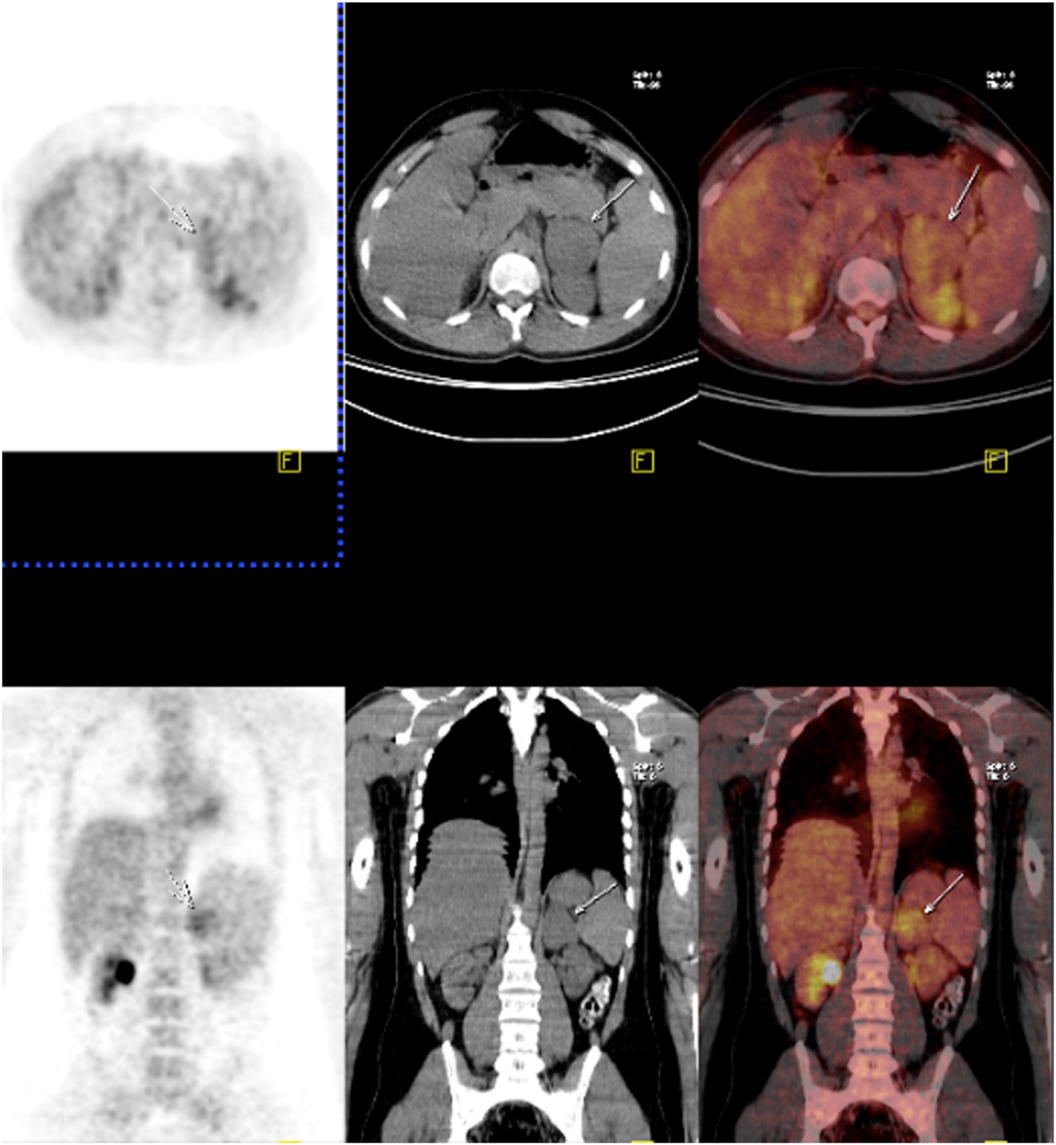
Positron emission tomography and computerized tomography scans of the patient with a left adrenal tumor. ^18^F-2-fluoro-deoxy-D-glucose-positron emission tomography scans show a standard uptake value of 4.1 for a left adrenal mass measuring 5.2×4.3×7.1cm. (arrow shows the mass on computed tomography).
